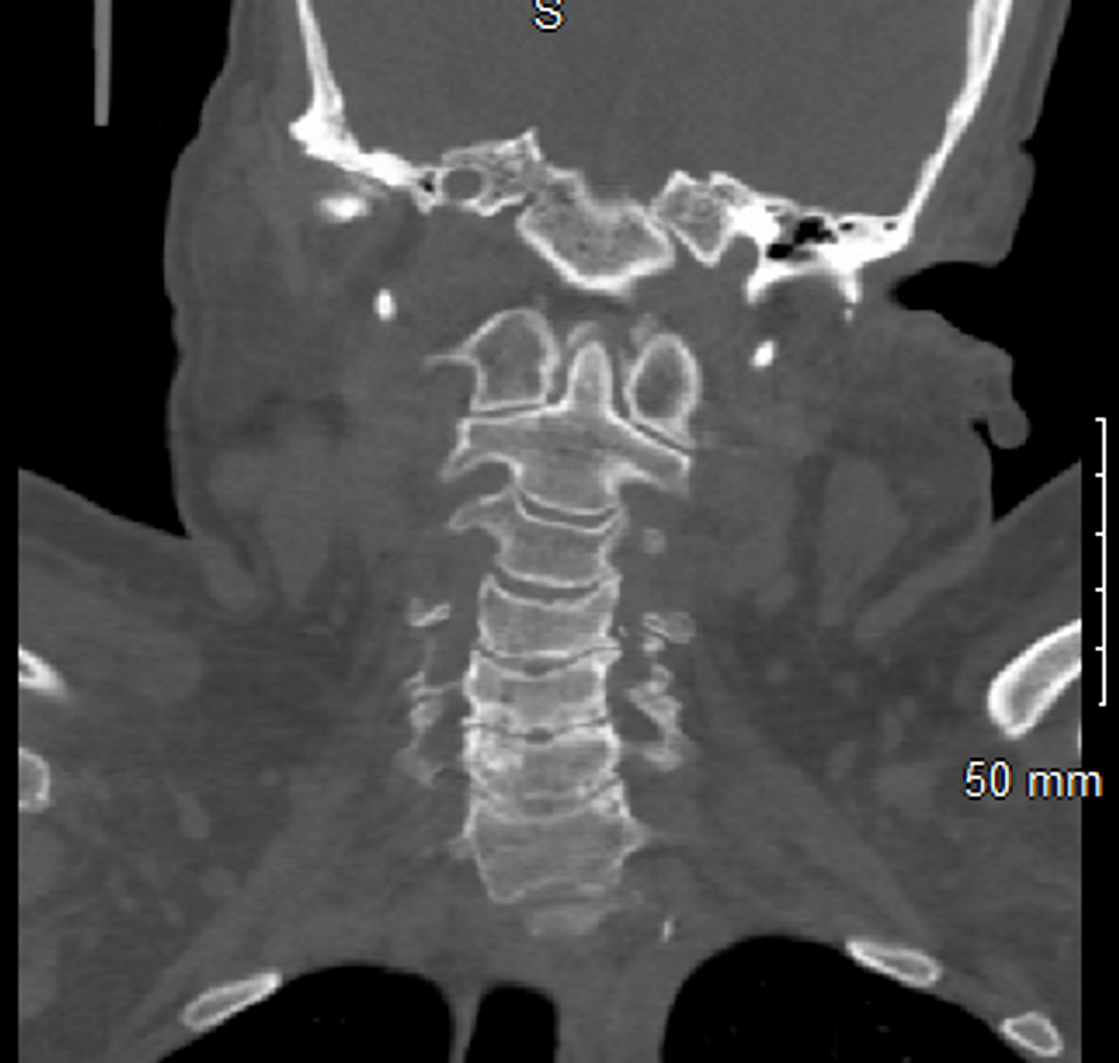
Cervical spine CT scan showing calcifications of the alar ligament (crowned dens syndrome)
